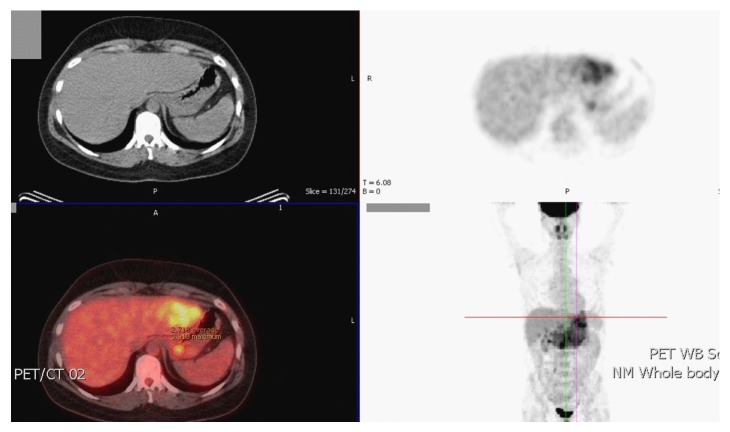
PET CT showing areas of increased ^18^FDG uptake correlating to lesions in segments two, three, and 4 of the liver.
